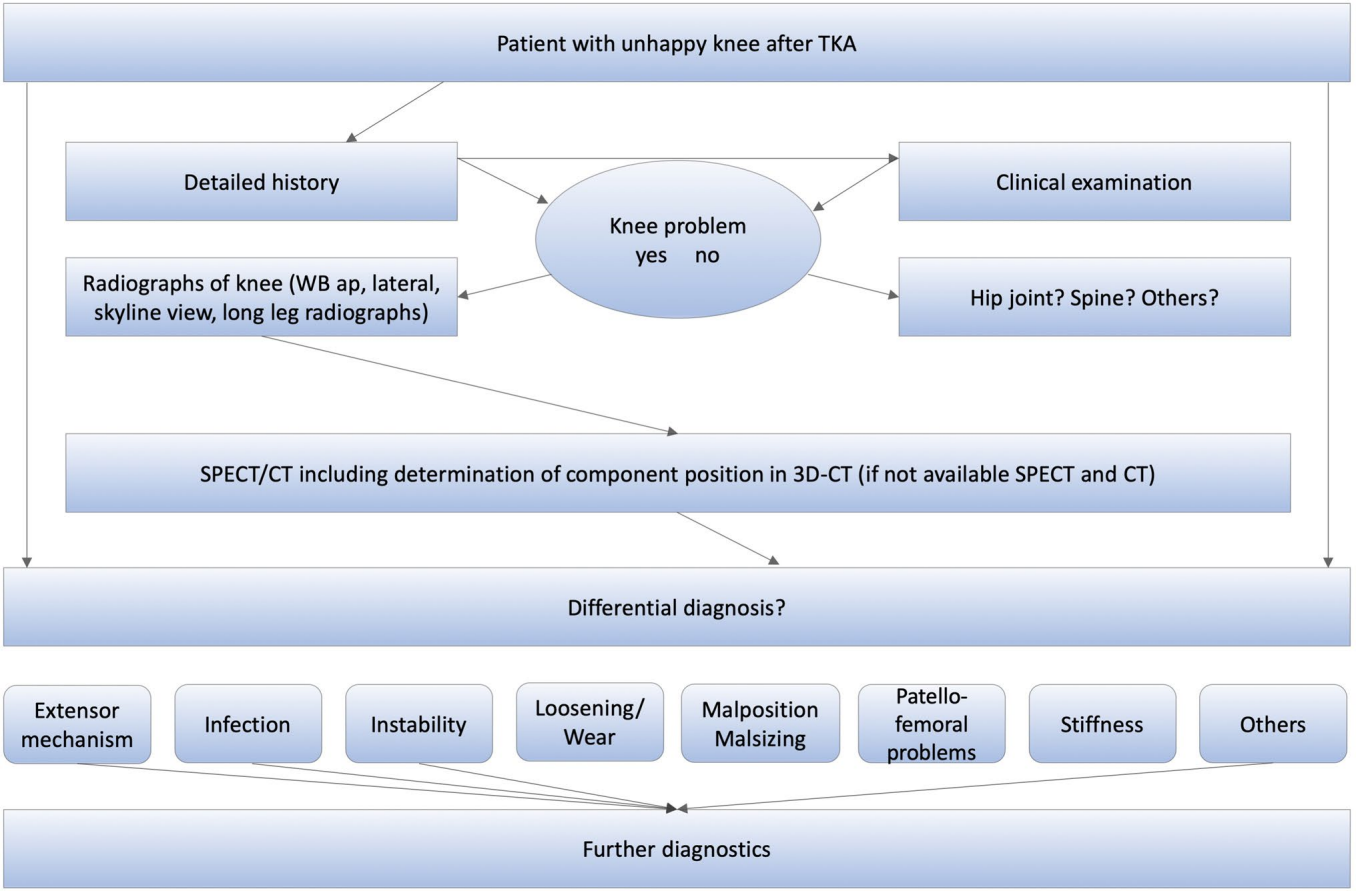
The “Bruderholz” standardized diagnostic algorithm for patients with pain after total knee arthroplasty. WB, weight bearing; SPECT/CT, single photon emission computed tomography/computer tomography. Reprinted with permission [25]
